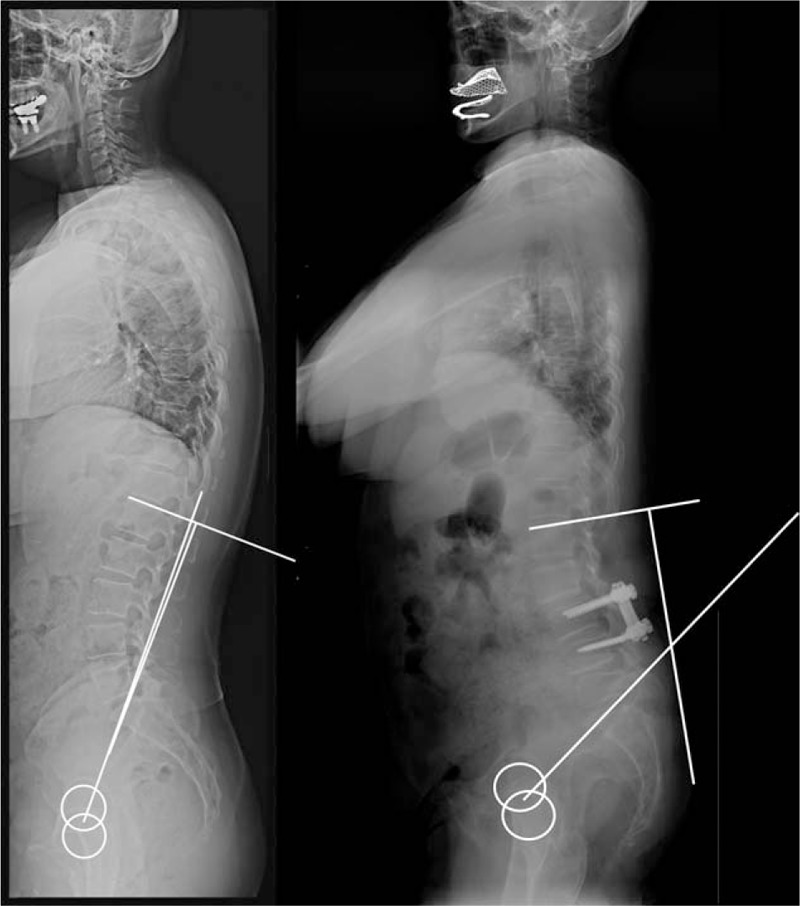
Comparison of a balanced spine and stooped spine according to L1 incidence. In a patient with a balanced spine, the L1I value is almost zero because LL is sufficiently compensated regardless of the amount of PI. On the contrary, in a stooped patient who has sagittal imbalance, L1I is increased in proportion to the PI/LL mismatch, and it is expressed in the whole-spine standing lateral radiograph. L1I = L1 incidence, PI = pelvic incidence, LL = lumbar lordosis.
